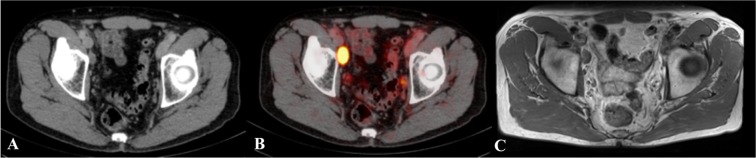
Example of a lymph node which is directly detectable on T1 MRI and ^68^Gallium-Prostate specific membrane antigen PET-CT. This figure shows a lymph node (LN) which is directly detectable on T1 MRI and ^68^Gallium-Prostate specific membrane antigen PET-CT. This large suspect LN is located at the right external iliac artery. The LN is visualized in corresponding axial plane slices using CT, ^68^Gallium-Prostate specific membrane antigen PET-CT and T1 sequence MRI presented left to right. (A) Lymph node depicted in CT, (B) Lymph node depicted in ^68^Gallium-Prostate specific membrane antigen PET-CT; (C) Lymph node depicted in the T1 sequence of MRI.
